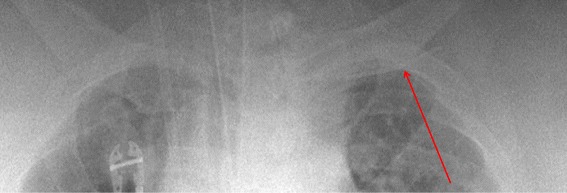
Portable AP Chest X-ray showing the fractured access needle within the left anterior chest wall.
